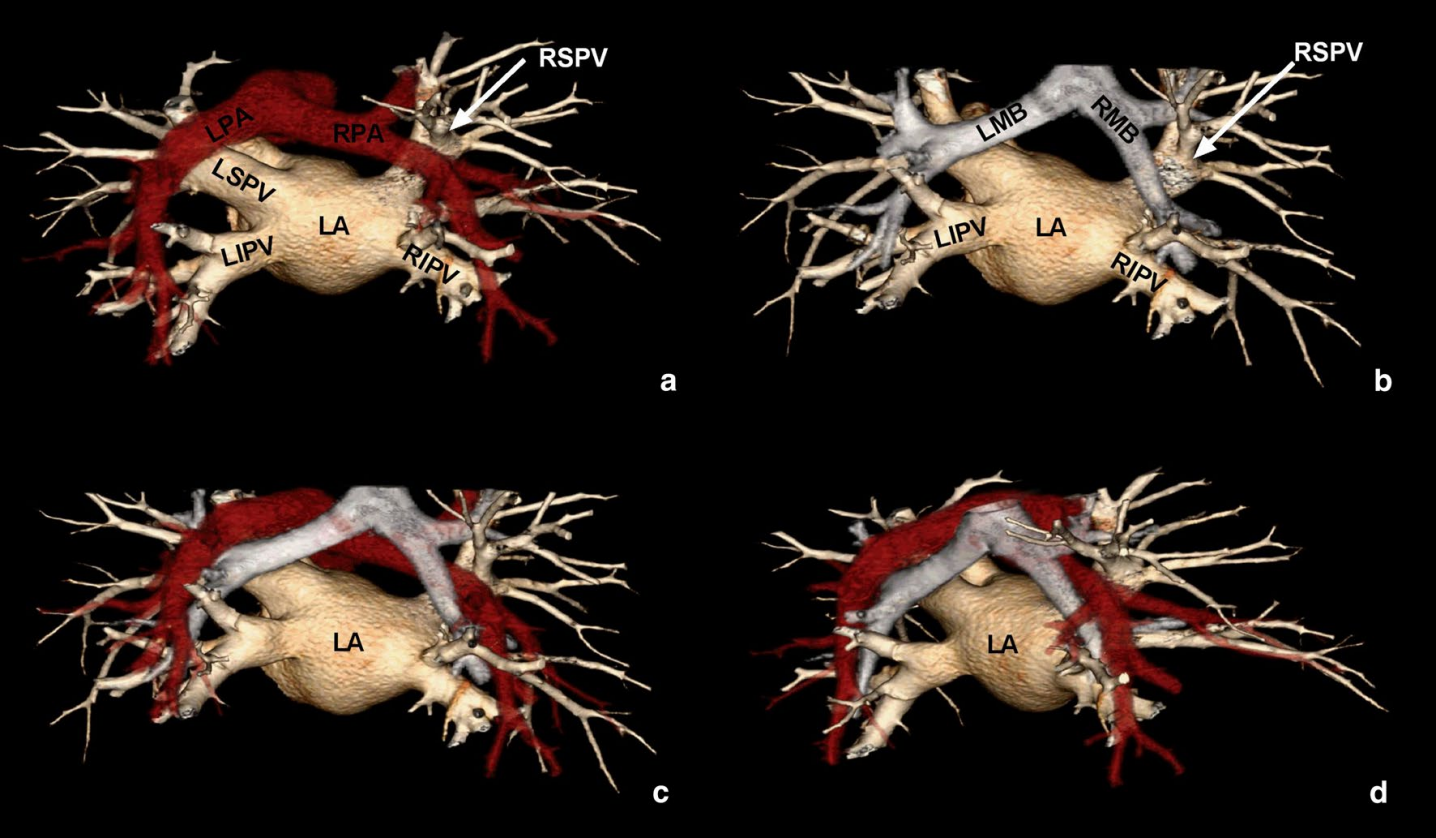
Three dimensional (3D) CT images of the left atrium (LA), pulmonary vein (PV), bronchial tree, pulmonary artery (PA), and branches. a 3D image of the LA, PVs and PAs. b 3D image of the LA, PVs, and bronchi. c, d 3D images of the LA, PVs, PAs and bronchi at different angles of view. LPA, left PA; RPA, right PA; RSPV, right superior PV; RIPV, right inferior PV; LSPV, left superior PV; LIPV, left inferior PV; RMB, right main bronchus; LMB, left main bronchus
